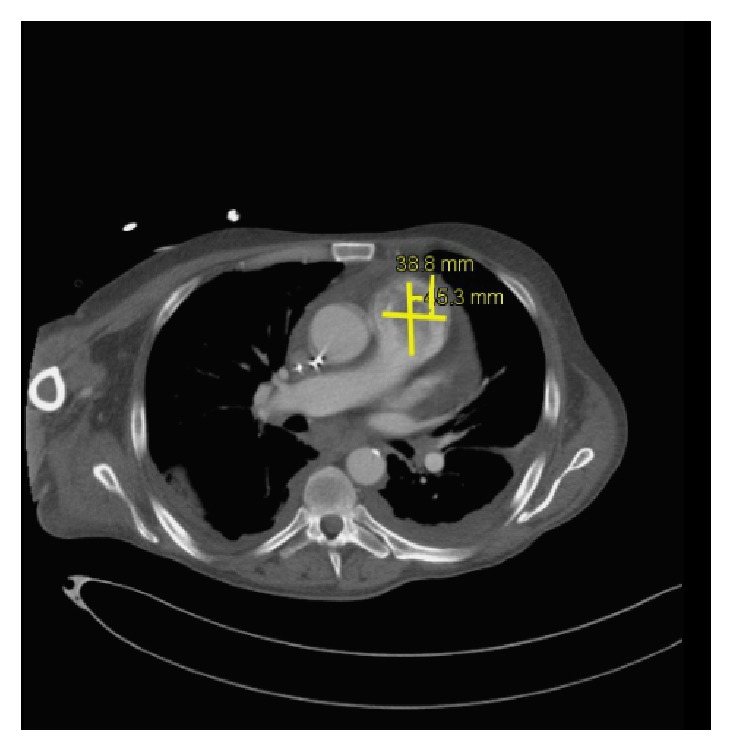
On the CT scan, the tumour is evaluated at 38.8 mm × 43.5 mm.
